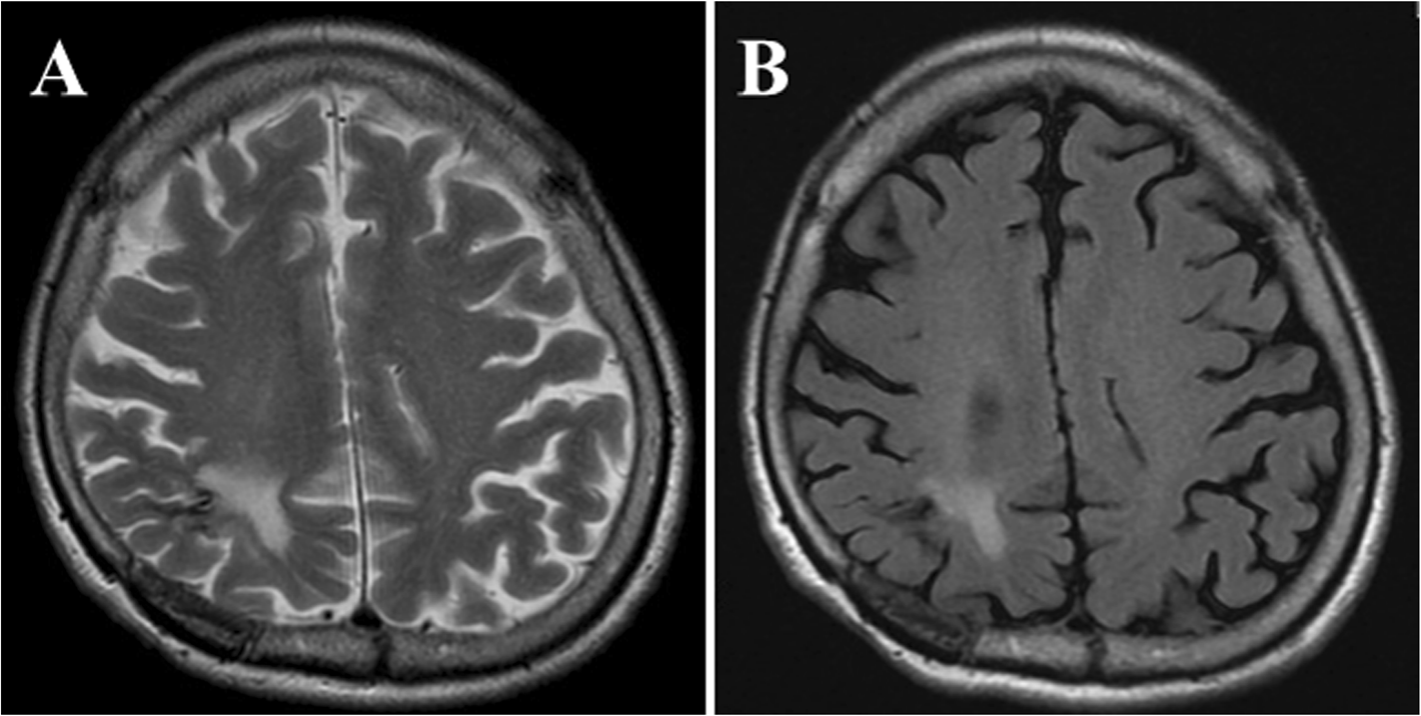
T2WI (a) and FLAIR (b) 4 years after admission revealed no new lesion
